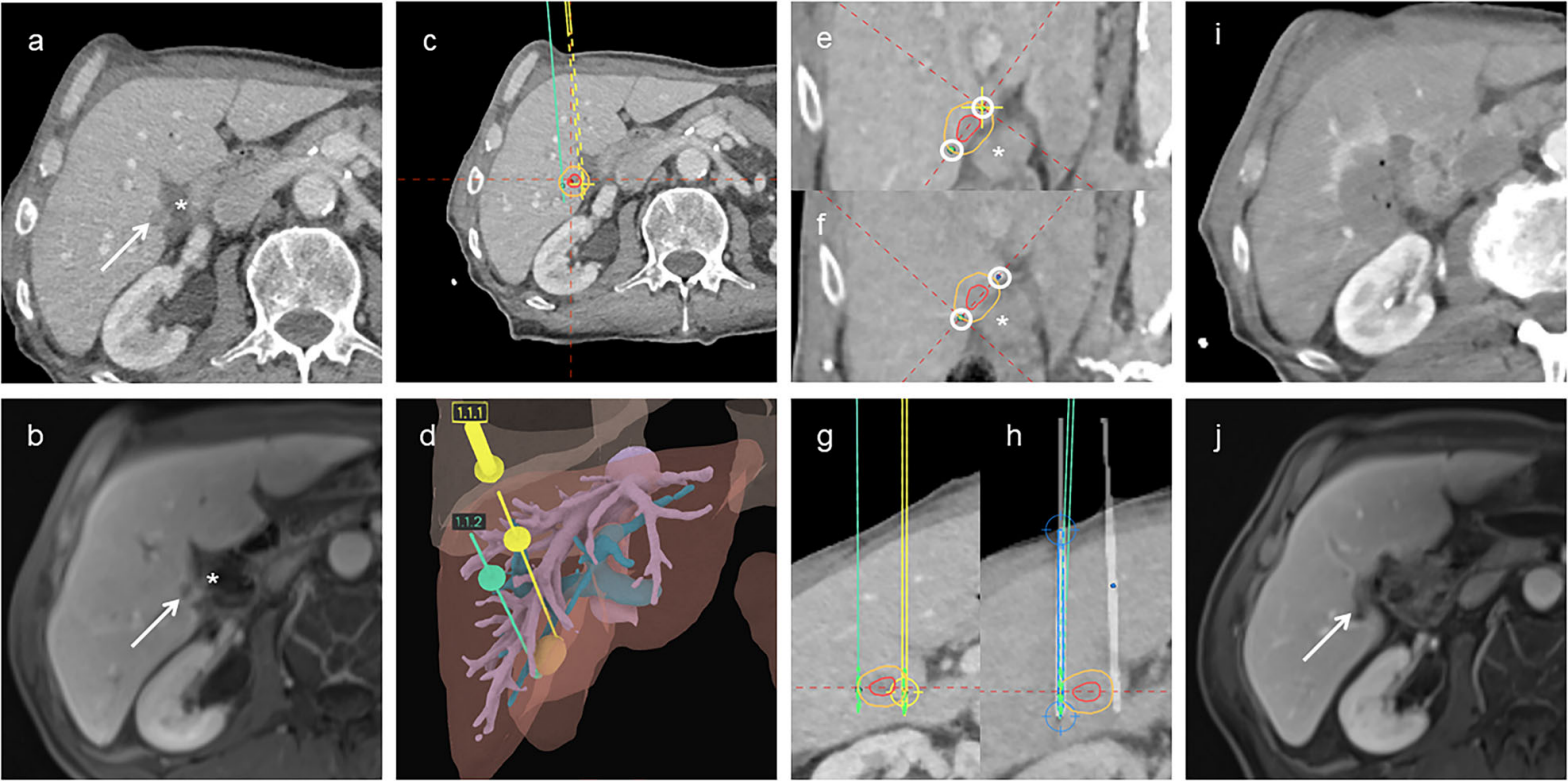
Stereotactic two-needle irreversible electroporation in a patient with a pancreatic metastasis near the duodenum. a, b Axial contrast-enhanced CT and MRI showing the tumor (arrow) adjacent to the duodenum (*). c Axial planning CT with planned needle trajectories; tumor (red) and 5-mm safety margin (yellow) outlined. d Three-dimensional liver rendering showing tumor (orange), hepatic veins (purple), portal vein (cyan), and planned needle paths. e Planning CT orthogonal to the needle axis with tumor (red), margin (yellow), and planned needle paths (circles). f Fused post-placement and planning CT confirming alignment of actual (circles) and planned needle paths. g Planning CT in a plane parallel to the needle axis, showing planned needle positions and active tip segments (green). h Fused CT confirming precise needle placement. i Immediate post-procedure CT showing complete ablation coverage. j Three-month follow-up MRI confirming complete ablation with a residual parenchymal defect (arrow). CT, Computed tomography; MRI, Magnetic resonance imaging
